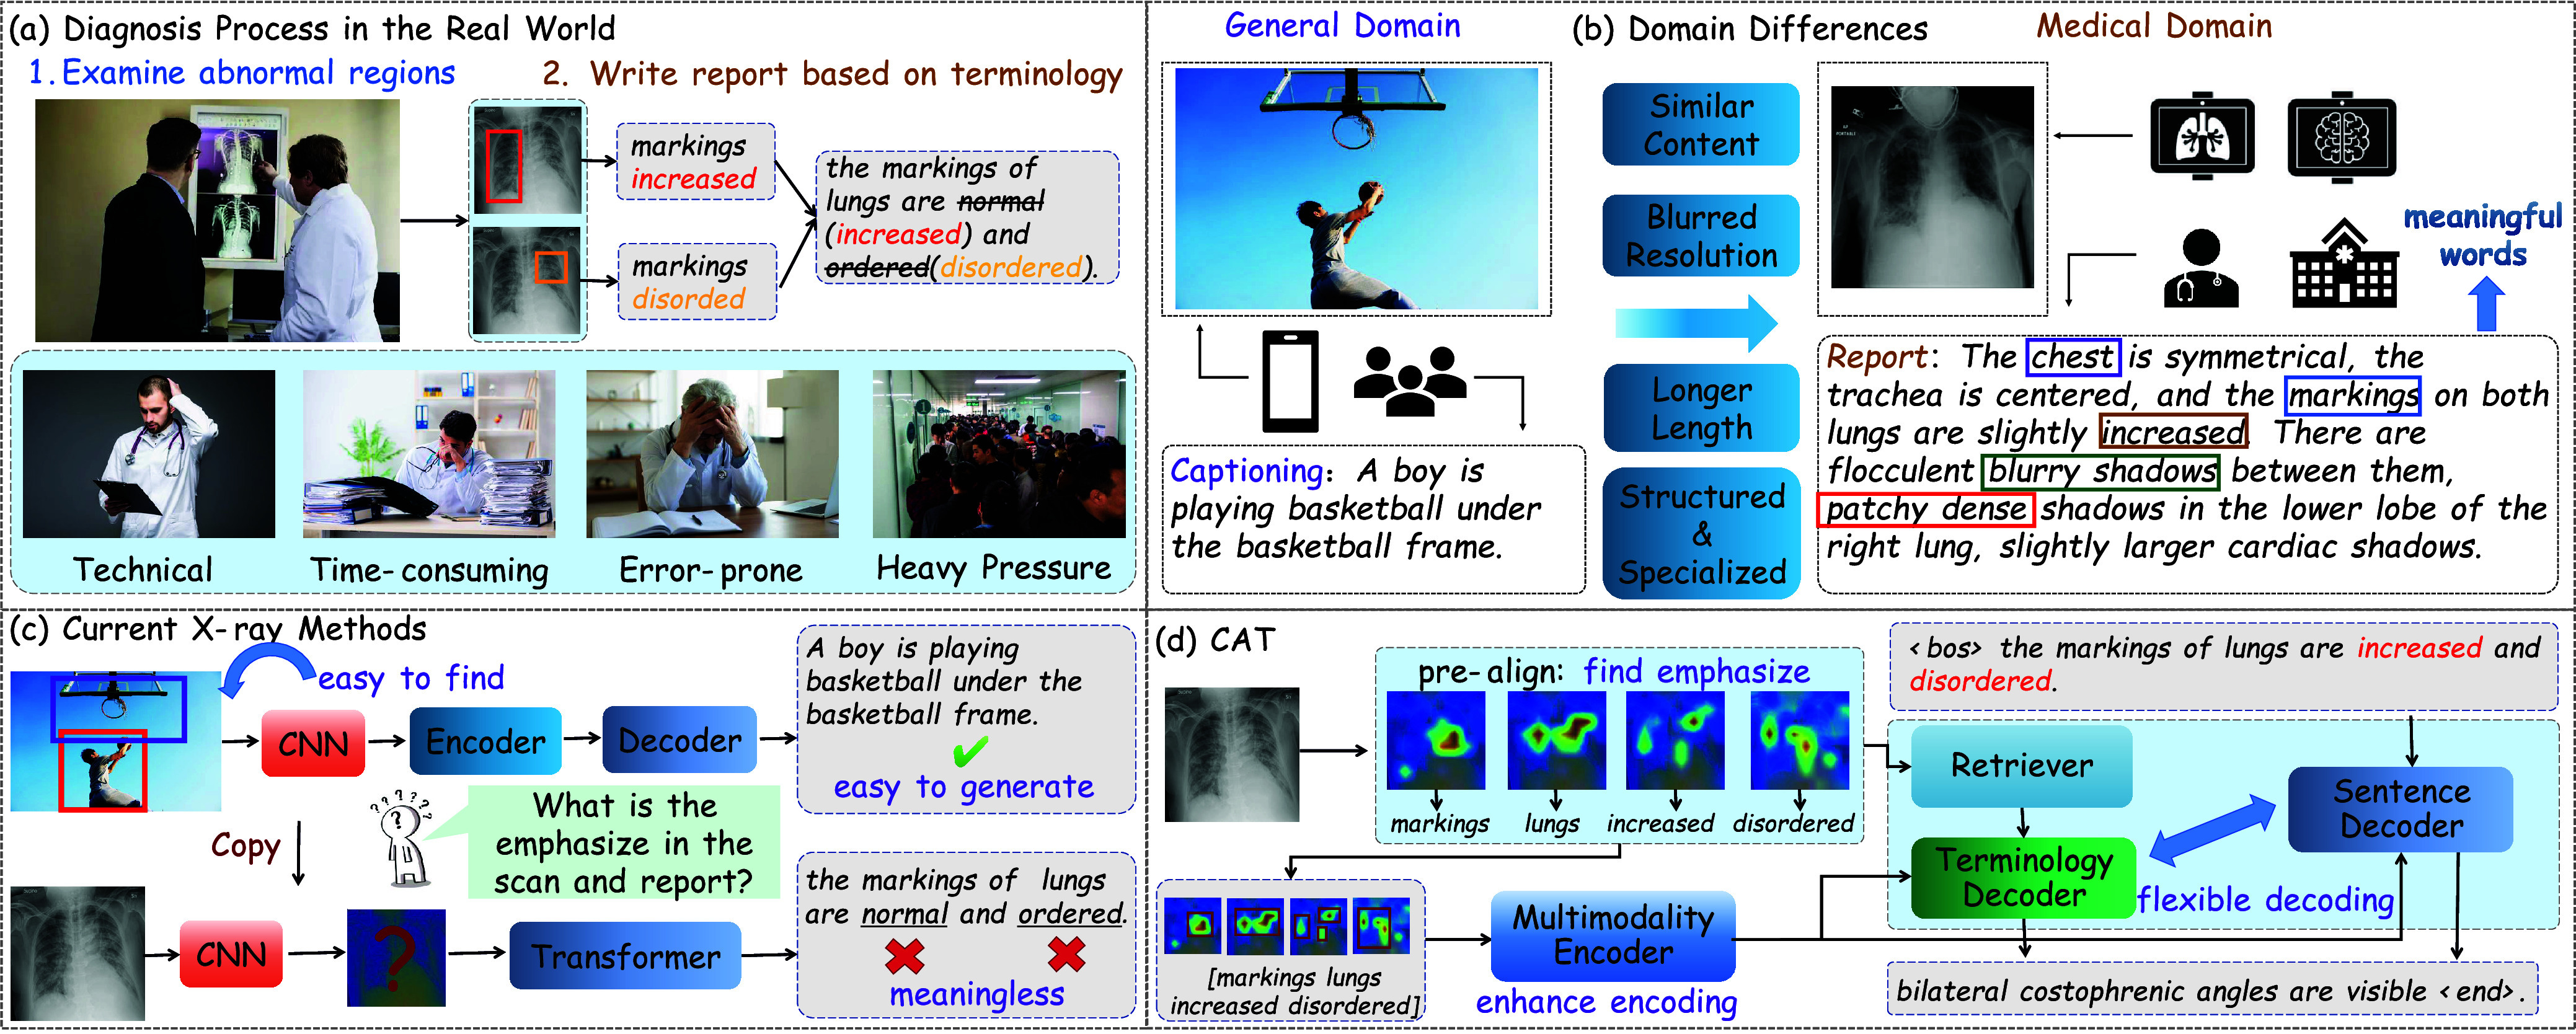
The current diagnostic process worldwide is not only time-consuming and labor-intensive but also susceptible to errors. Medical datasets often exhibit notable data biases, and existing X-ray methods may fail to capture rare yet significant abnormalities, resorting instead to generating template sentences. In contrast, the proposed model surpasses baseline methods that only produce normal descriptions by generating more comprehensive abnormal descriptions.
